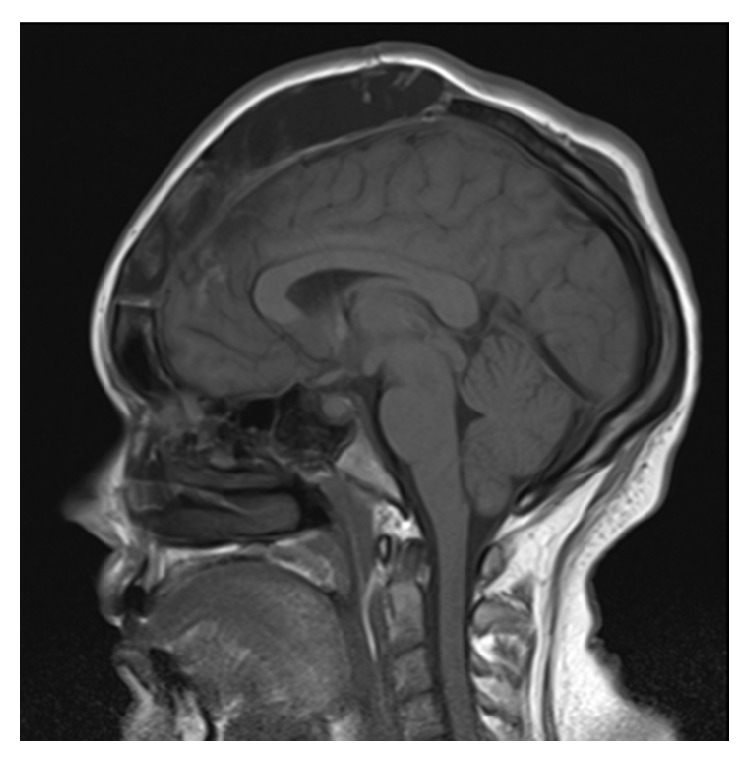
Sagittal MRI 3 weeks after decompressive craniectomy.
